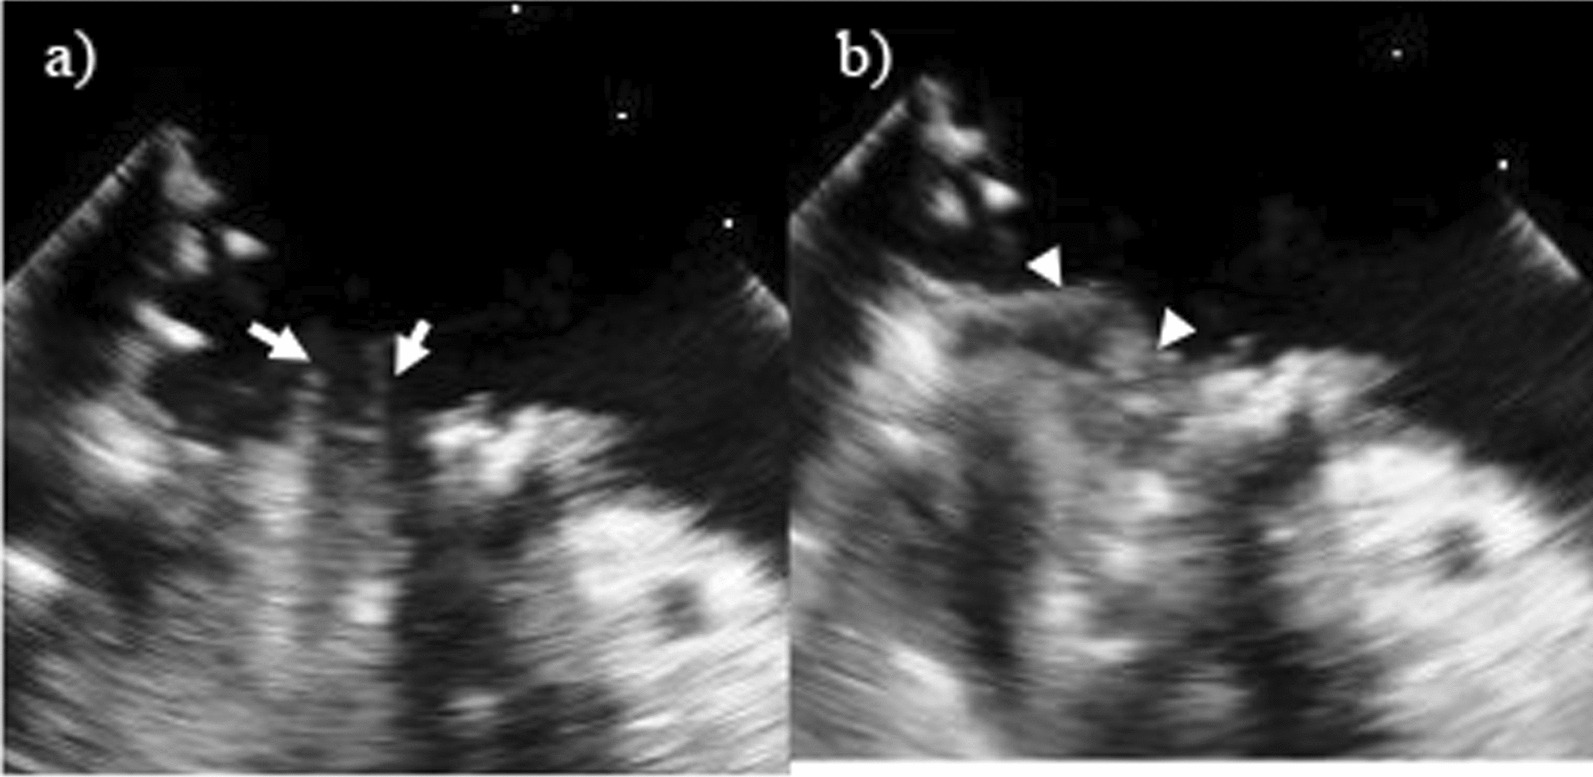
Postoperative transesophageal echocardiography. Postoperative transesophageal echocardiography reveals that the mitral prosthetic valve is functioning normally at both the a open (white arrows) and b closed (white arrowheads) positions, without perivalvular leakage
